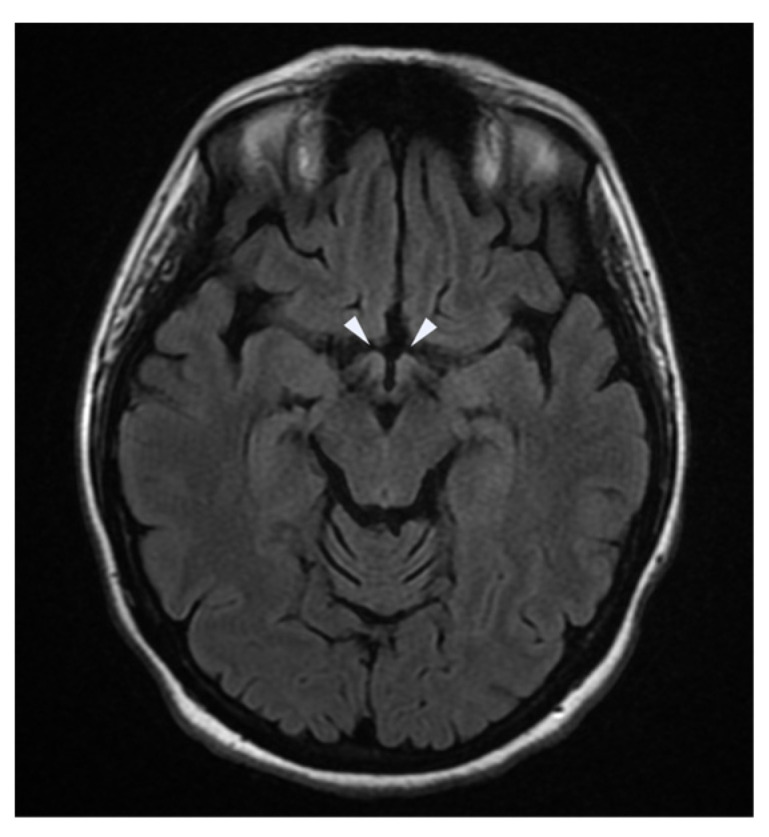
FLAIR axial view of the mammillary bodies.
